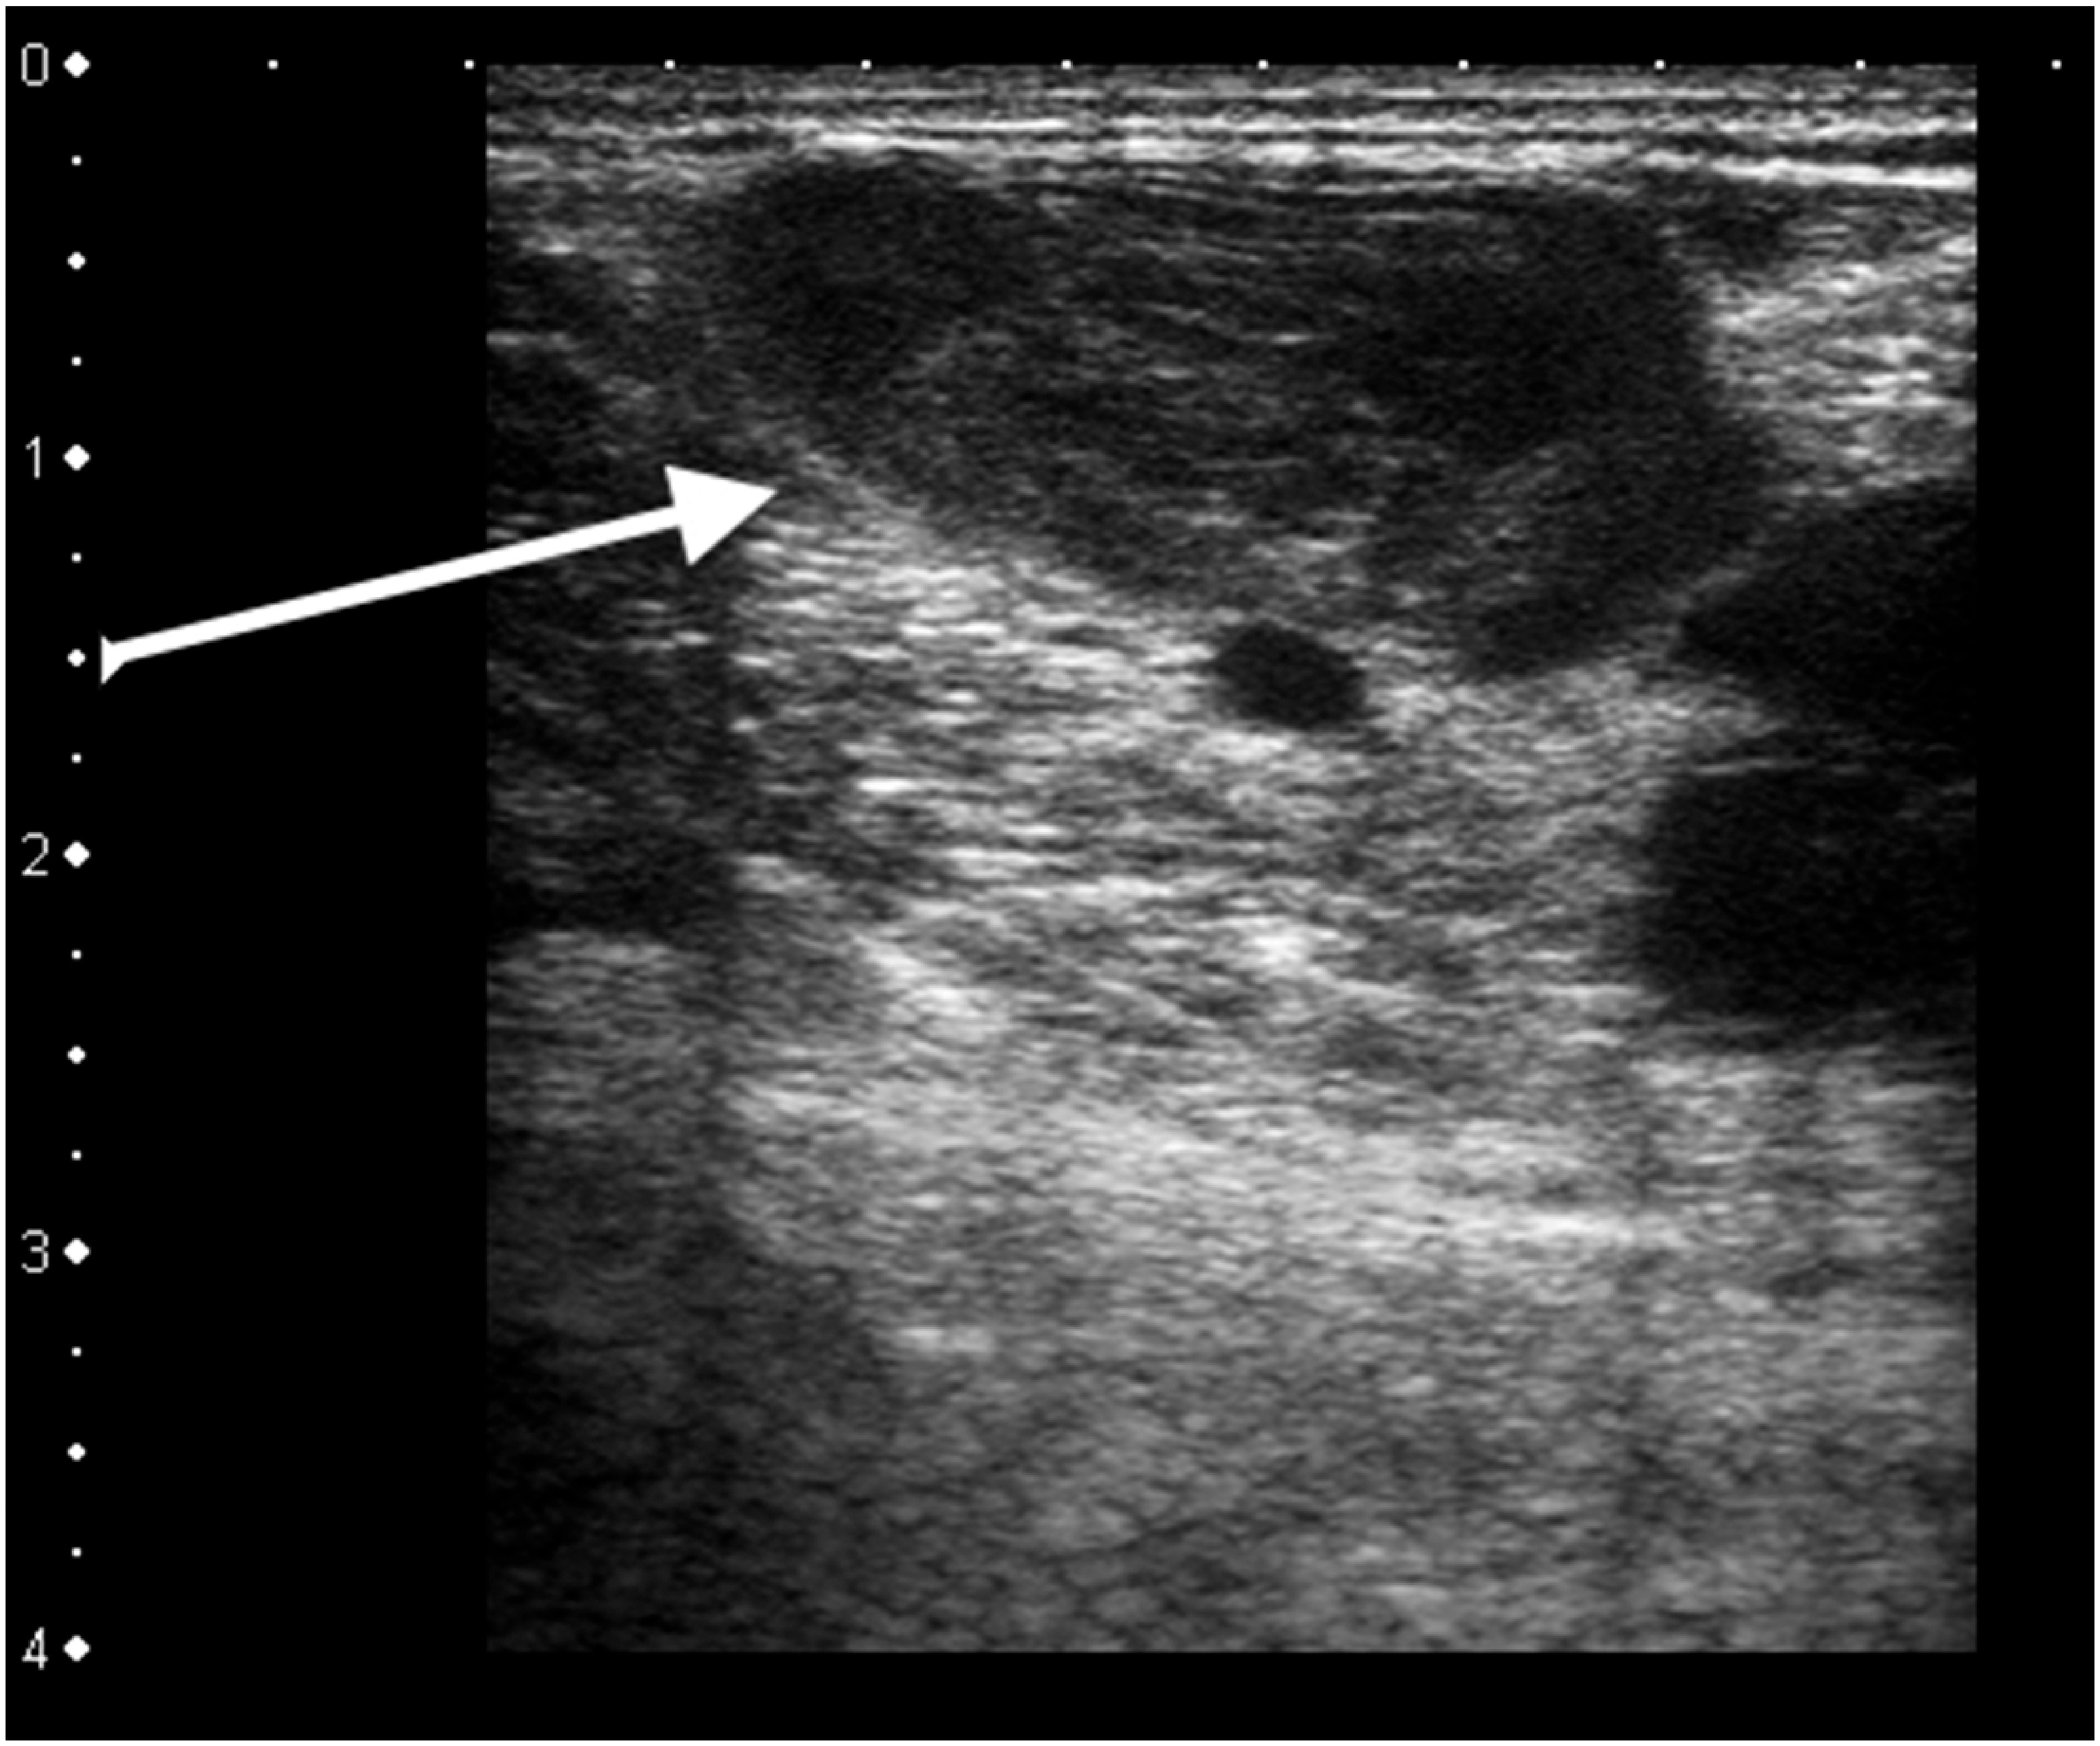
Fig. 1 Preoperative duplex ultrasonography showed a 24.3 mm ×20.4 mm saccular-type aneurysm in the sural vein with thrombus (white arrow).
